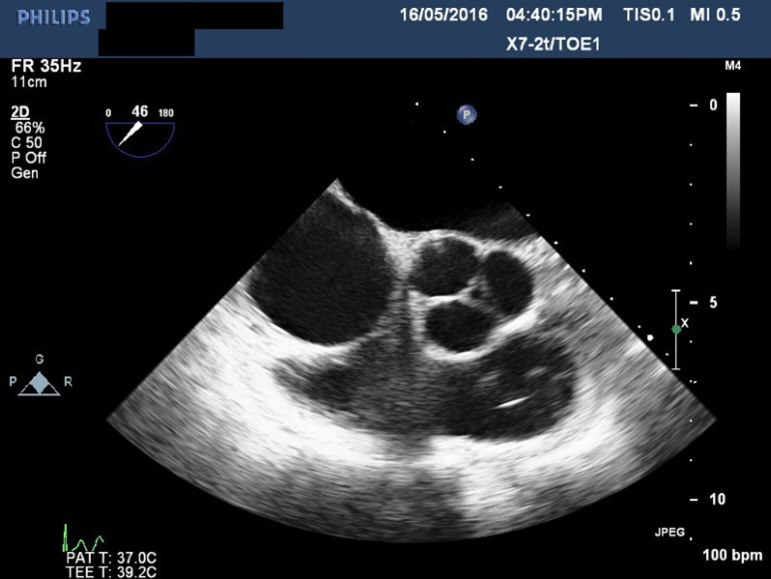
Transesophageal echocardiogram showing thickened aortic valve
